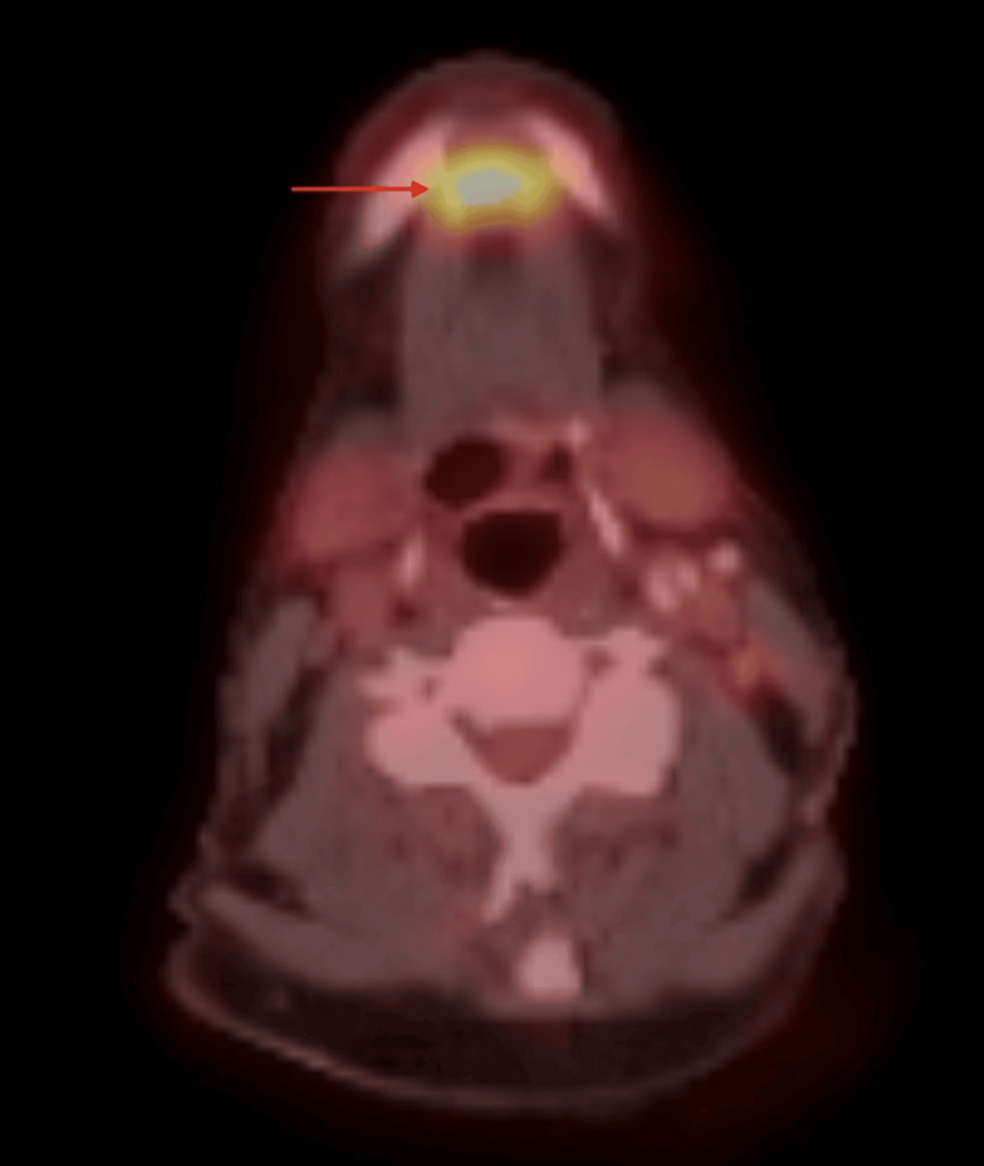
Axial PET/CT Neck of Mandibular Mass Axial PET/CT neck showing a FDG avid mass in the mandibular symphysis (red arrow)
